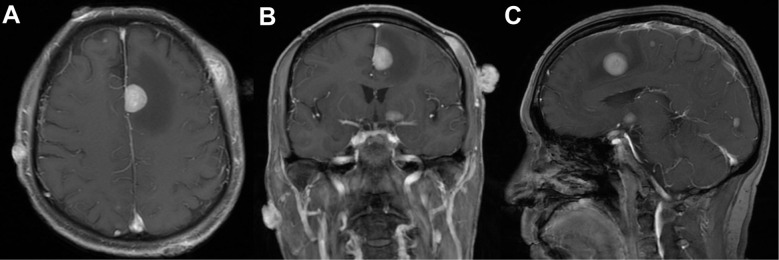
Magnetic resonance imaging of the brain on August 24, 2023 demonstrate numerous heterogeneously enhancing intracranial lesions, suggestive of brain metastases. (A) Axial, (B) coronal, and (C)​ sagittal fat-suppressed, contrast-enhanced T1-weighted images.
